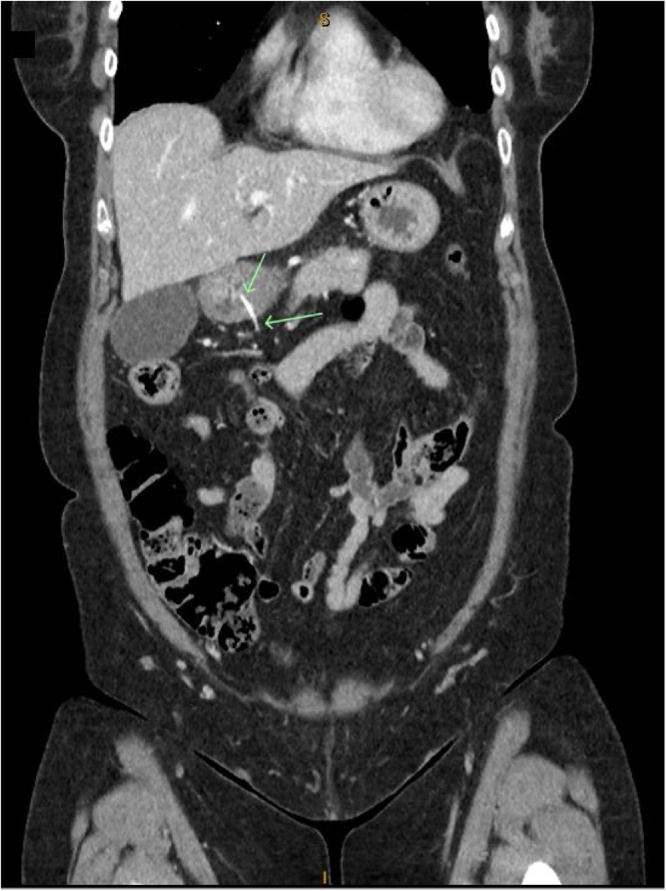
CT abdomen revealed a calcified foreign body perforating the gastric wall.
